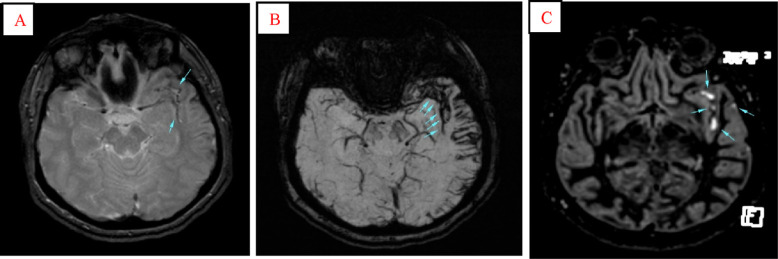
Spontaneous subarachnoid haemorrhage in a 44-year-old woman. MR images were performed 18 days after injury. Using T2*-W (A, arrows) and SWI (B, arrows) images, detection of SAH is a challenging issue due to its adjacency to main veins in the base of the skull and the similarity of signal intensity between these veins and haemorrhage may lead to misdiagnosis of SAH in this region of brain. In DIR image (C, arrows), SAH is demonstrated as marked several hyper-intense regions in the left Sylvian cistern. Note that detecting SAH in this area is one the priorities of DIR sequence to T2* and SWI.
